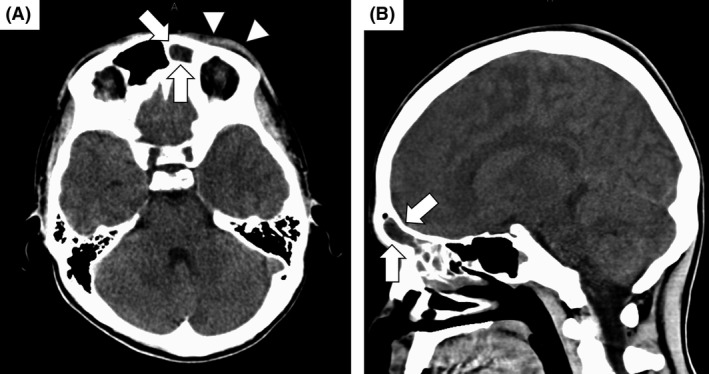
Computed tomography (CT) images of the head on the day of admission. (A and B) Attenuation of the tissue within the left sinus is observed (arrows). Periorbital soft‐tissue thickening is also observed (arrow heads). Bone abnormalities and continuity with the intracranial space are not observed. Intracranial subdural abscess is not apparent on CT images.
